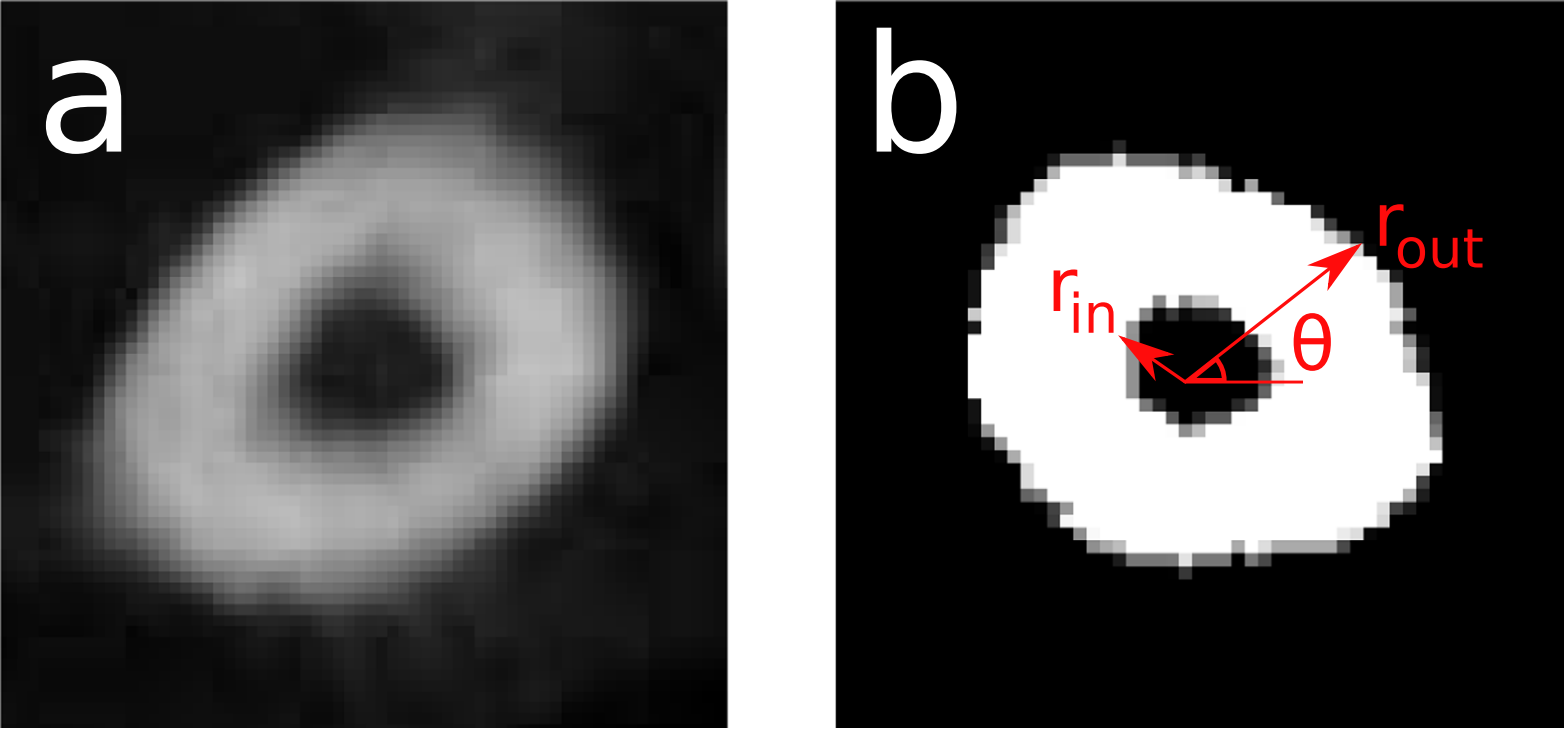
(a) Example of an original *μ*CT-slice at height *z*′ = *ξ*. (b) The orthogonal slice at height *z*′ = *ξ* with the outer and inner radii marked. These defined the thickness of the cortical bone, Ψ(*ξ, θ*), by taking the difference between the radii.
